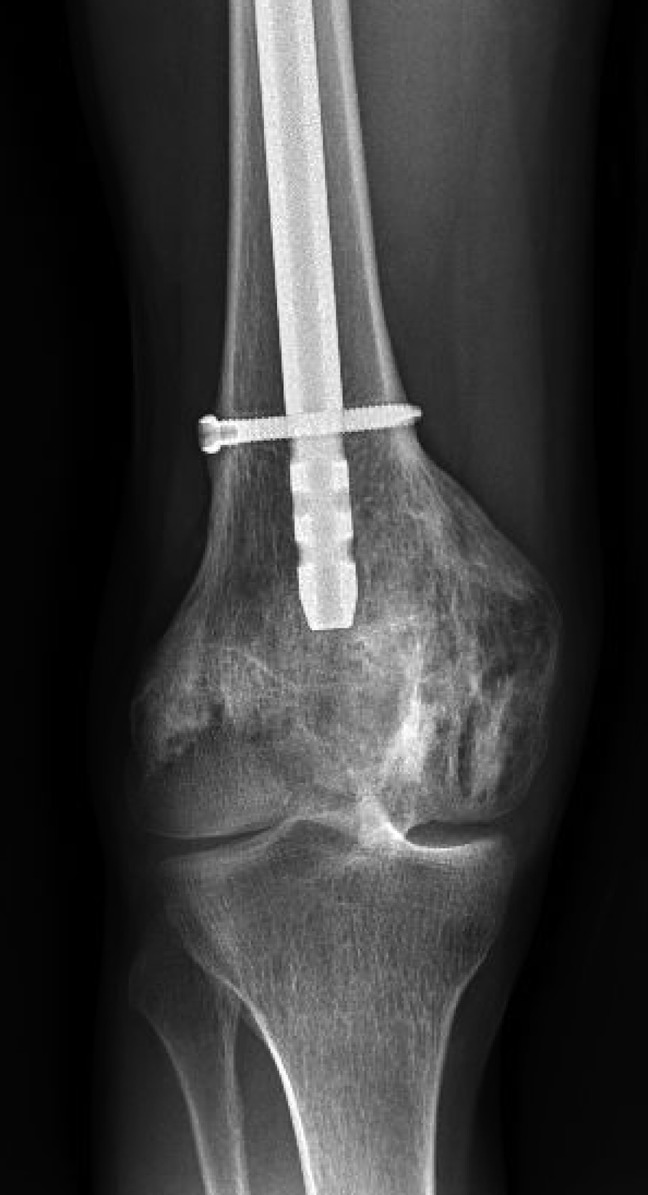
A radiograph 2 years before presentation showing a nailed right mid femoral shaft fracture and healed medial femoral condyle fracture with increased sclerosis of the brown tumor.
